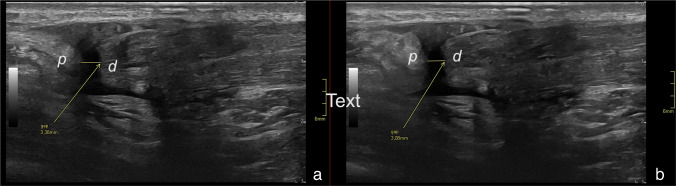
Ultrasound long-axis image demonstrating concordant tendon gap measurement between two raters. Both raters identified similar tendon stump margins and placed calipers in comparable positions, resulting in similar gap measurements (a-Rater 1: 3.4 mm; b- Rater 2: 3.1 mm). The measurements fall on the same side of the 5—mm threshold, leading to consistent classification within the treatment pathway. p = proximal stump; d = distal stump
